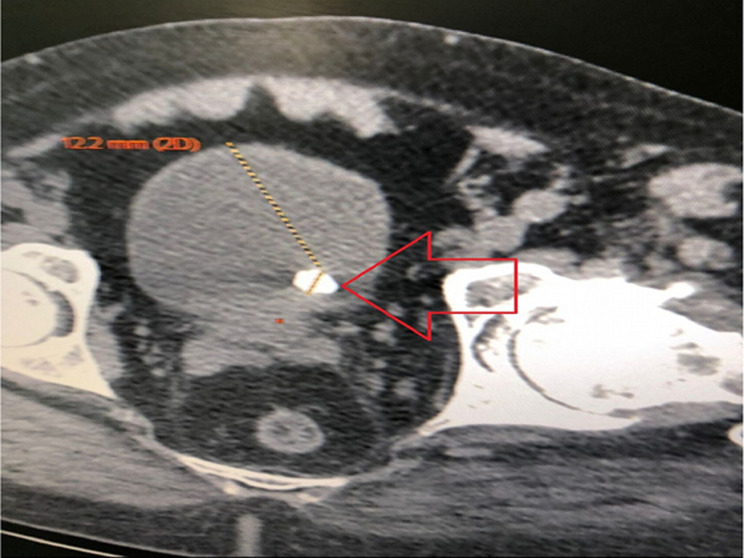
computed tomography scan showing a bladder stone with wall thickening of the bladder
